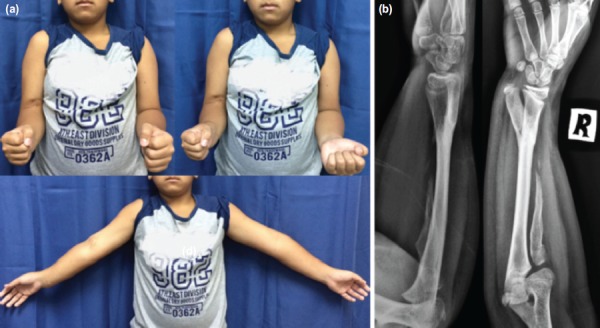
(a) Photographs of the patient at 12 months post-operatively, showing loss of right forearm rotational movement and position of upper limbs with elbow in extension. (b) Forearm radiograph showing solid union at the fusion site.
